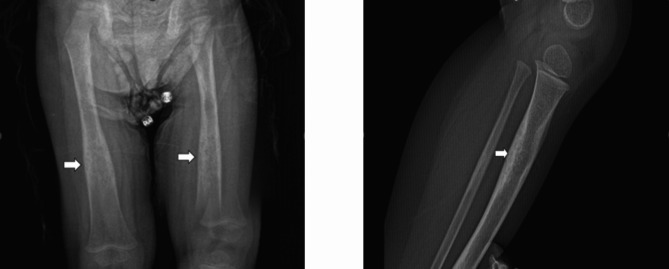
Radiography of the lower limbs shows cortical thickening of both femurs and tibia
